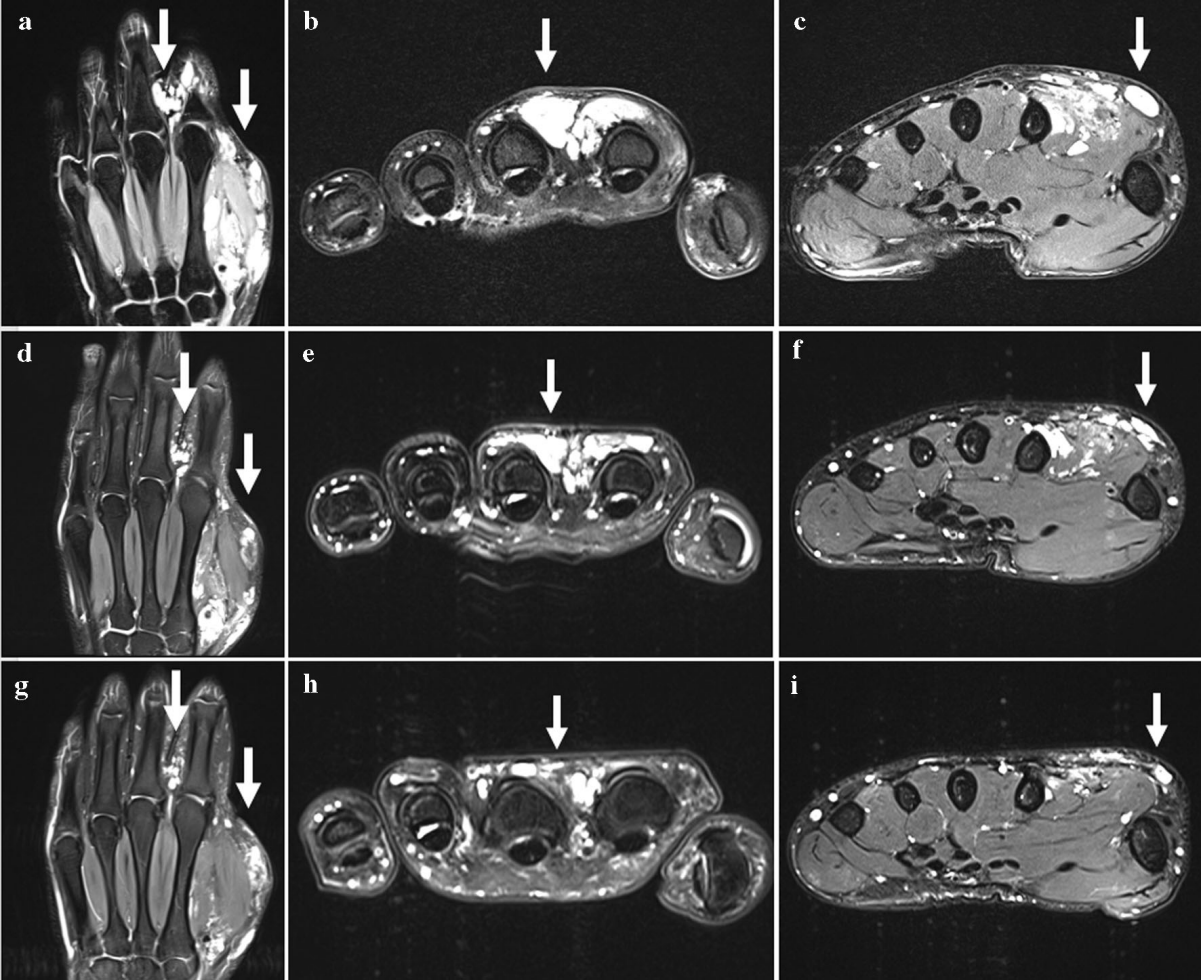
T1-weighted fast spin-echo fat-saturated MR images showing the hyperintense VM of a 23-year-old male patient at intervals between several sclerotherapy sessions. a-c Coronar and axial MR images demonstrate hyperintense tubular structures involving thenar and interdigital region of the left hand extending out to the dermis (arrows). d-f 4 months after treatment initiation: coronar and axial MR images reveal lesion size reduction (arrows) when compared to (a-c). g-i 9 months after treatment initiation: coronar and axial MR images show further lesion size reduction up to 50% (arrows) when compared to (a-c) and (d-f)
